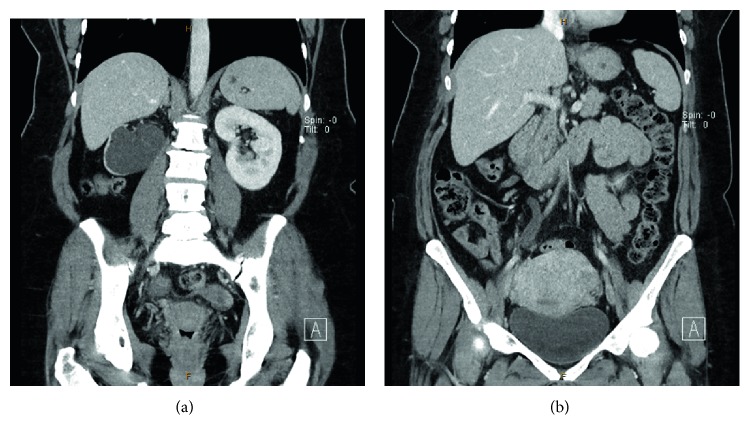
CT urogram confirmed significant right hydronephrosis with marked thinning of the renal parenchyma (a), and right hydroureter at the level of the pelvic brim where it crosses the common iliac artery (b).
